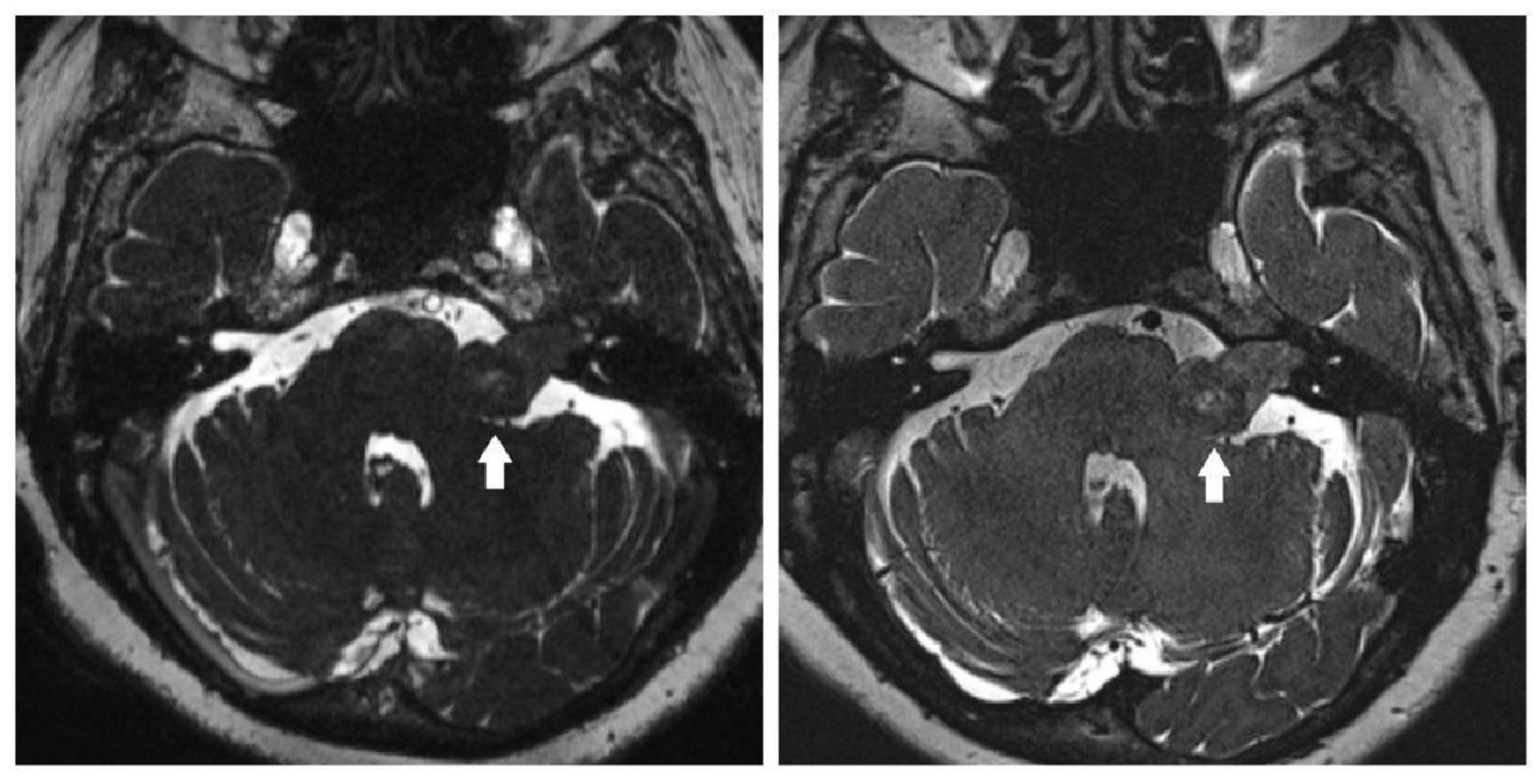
Axial T2-weighted 3D-CISS (a) and (b) 3D-SPACE images demonstrate left cerebellopontine angle schwannoma extending into the internal auditory canal. The signal quality of the lesion and indentation of the adjacent parenchyma is more pronounced on the 3D-SPACE sequences than the 3D-CISS sequences (arrows).
